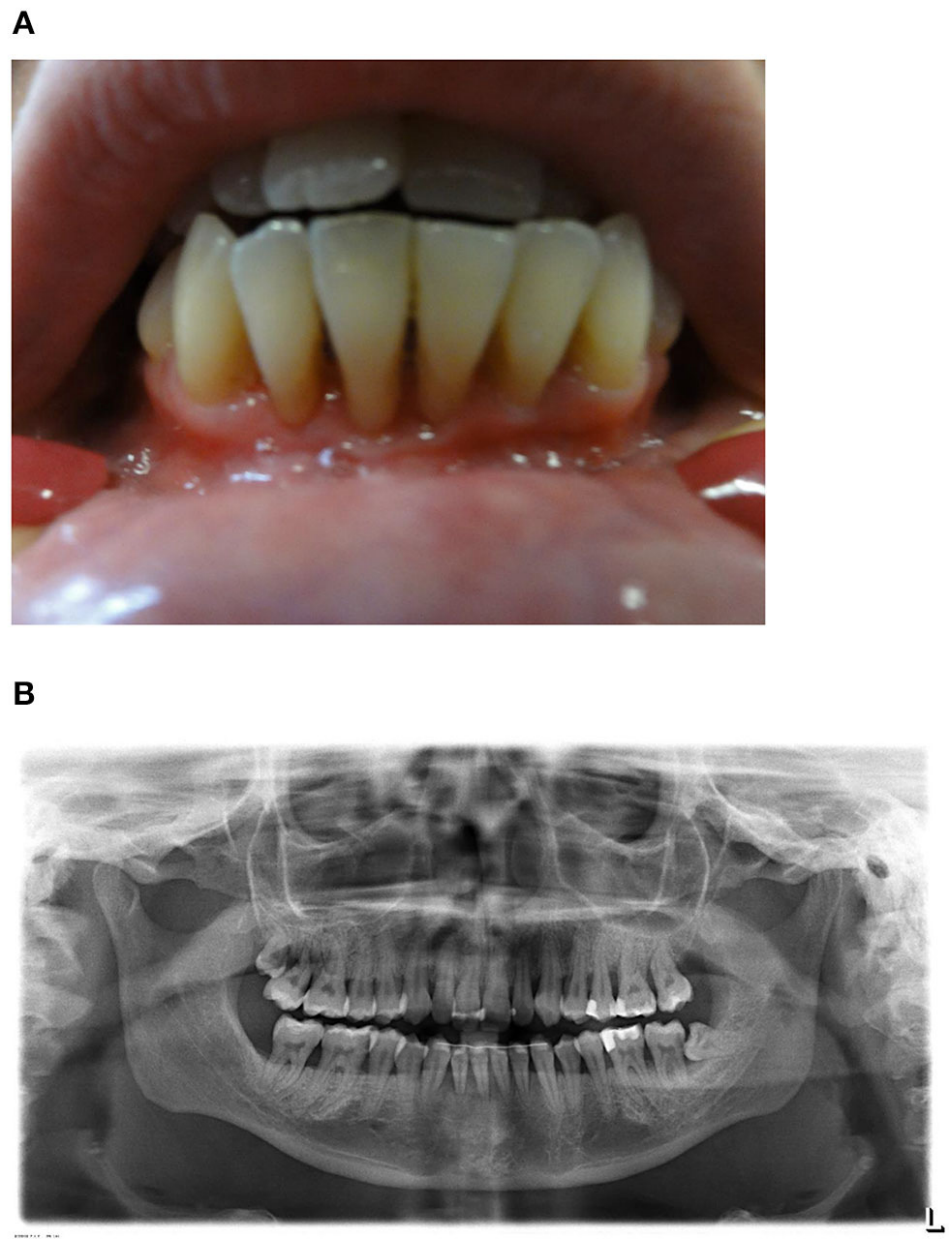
(A) Clinical findings. Exposed root surfaces of the teeth 33–43 due to severe gingivitis and periodontitis in an age of 24 years. (B) Orthopantomogram. General alveolar bone loss caused by severe chronic periodontitis. The wisdom teeth 18 and 38 are partially erupted and the mandibular anterior teeth are stabilized by a retainer.
